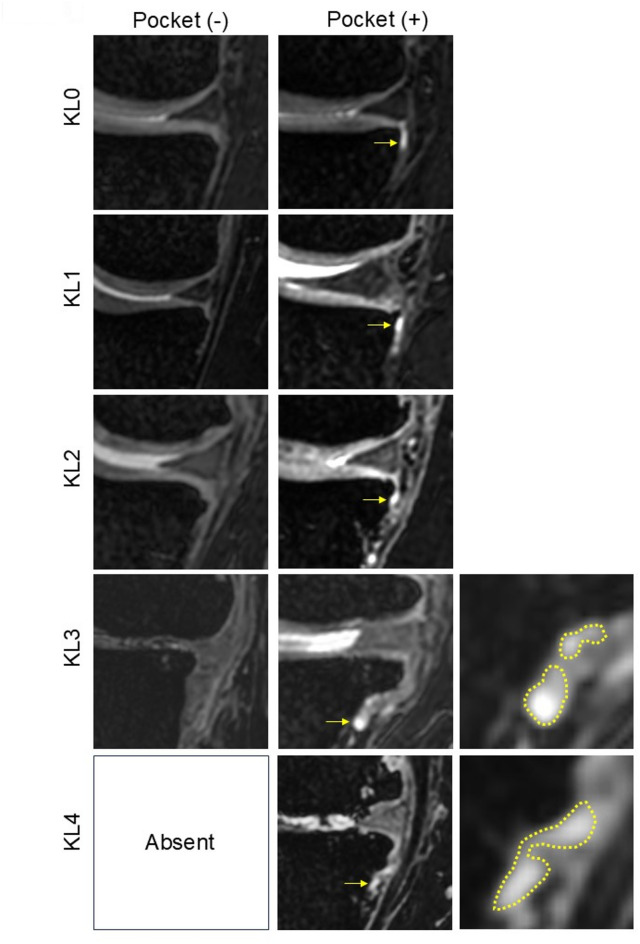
MRI appearance of the pocket sign across KL grades. Coronal SPGR MRI images of the medial compartment show knees without the pocket sign (pocket [−]) and with the pocket sign (pocket [+]) across KL grades. Yellow arrows indicate the characteristic pocket sign, defined as a focal fluid-equivalent signal at the meniscotibial ligament–bone interface. Enlarged images of pocket-positive KL grade 3 and KL grade 4 knees are shown in the rightmost column. In these enlarged images, the region classified as the pocket sign is outlined by a yellow dotted line. The panel labeled “Absent” indicates that no representative pocket-negative knee was available for KL grade 4.
